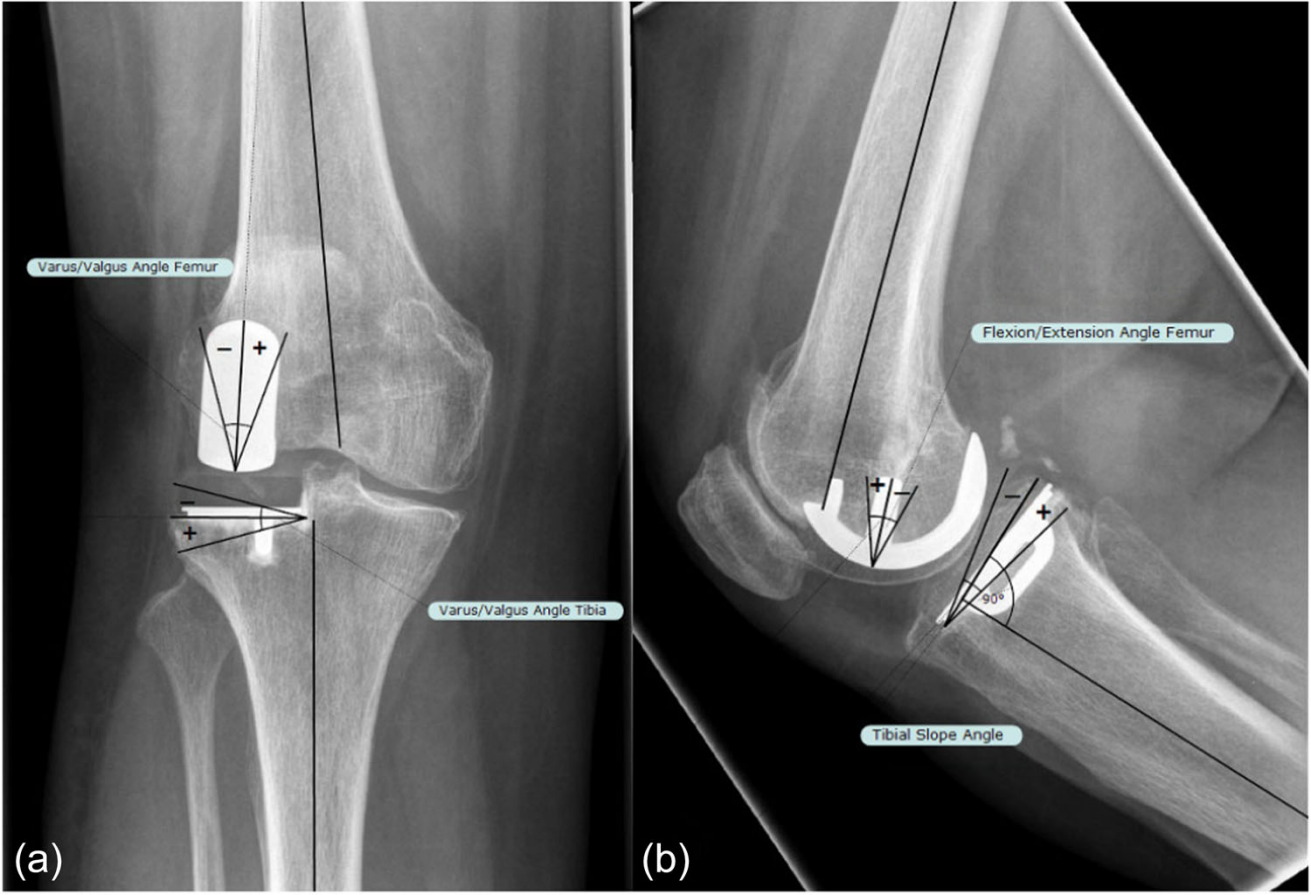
Radiographic assessment of femoral and tibial component alignment: (a) varus/valgus angle of femoral and tibial components on anteroposterior (AP) radiograph and (b) femoral flexion/extension angle and tibial slope angle on lateral radiographs. Positive values (+) indicate valgus and flexion for the femoral component, and valgus and posterior slope for the tibial component.
